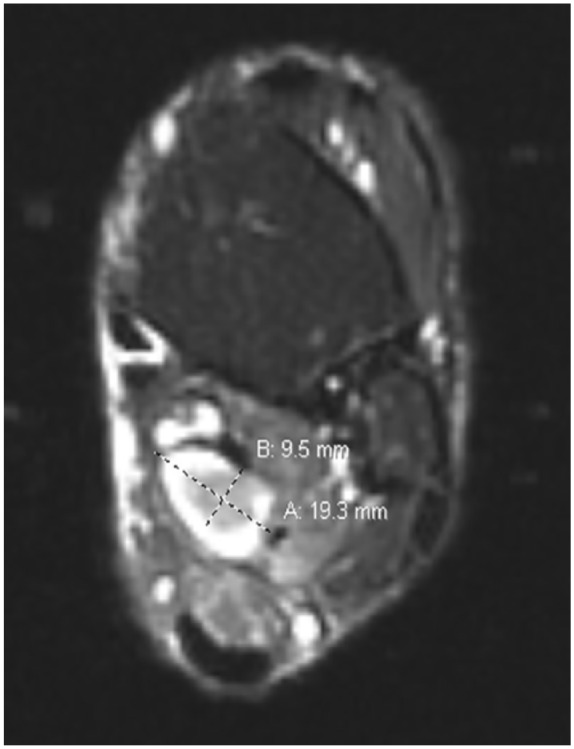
Axial T2 MRI image showing a well-defined hyperintense tibial nerve lesion measuring 9.5 mm × 19.3 mm.
